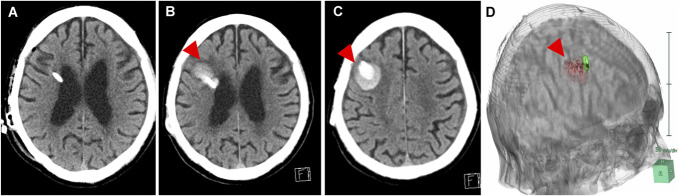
An example case of seizure due to intracerebral hemorrhage associated with ventricular shunt approximately 2 years after VA shunt surgery. A, CT scan immediately post-VA shunt placement. B and C, CT showed intracerebral hemorrhage around the ventricular shunt tube at approximately 2 years after VA shunt surgery. D, 3D reconstruction of CT images, red: hemorrhage, green: shunt tube. Arrowheads indicate the ventricular puncture site and the hemorrhagic lesion. VA, ventriculoatrial.
